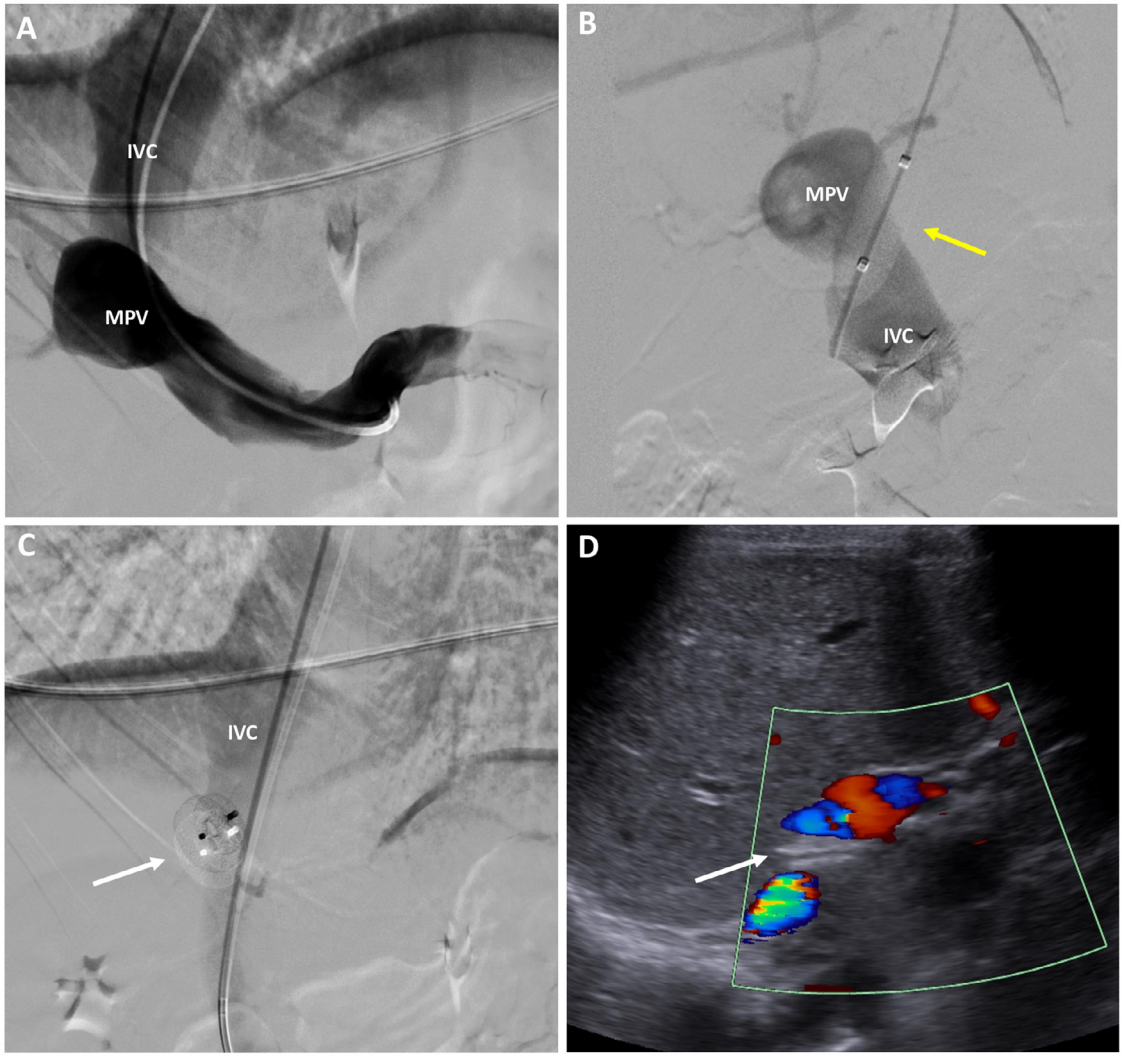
Transjugular portal venography and Doppler ultrasound. (A and B) Diagnostic venography with temporary shunt closure with a balloon (yellow arrow) shows opacification of the IVC, MPV and hypoplastic intrahepatic portal branches. (C) Therapeutic venography with deployment of a closure device (white arrow). (D) Color Doppler ultrasound performed 6 mo after device deployment demonstrates the device in the previous location of the portosystemic shunt (white arrow) and absence of flow between the MPV and IVC. IVC, inferior vena cava; MPV, main portal vein. “Color version available online.”
